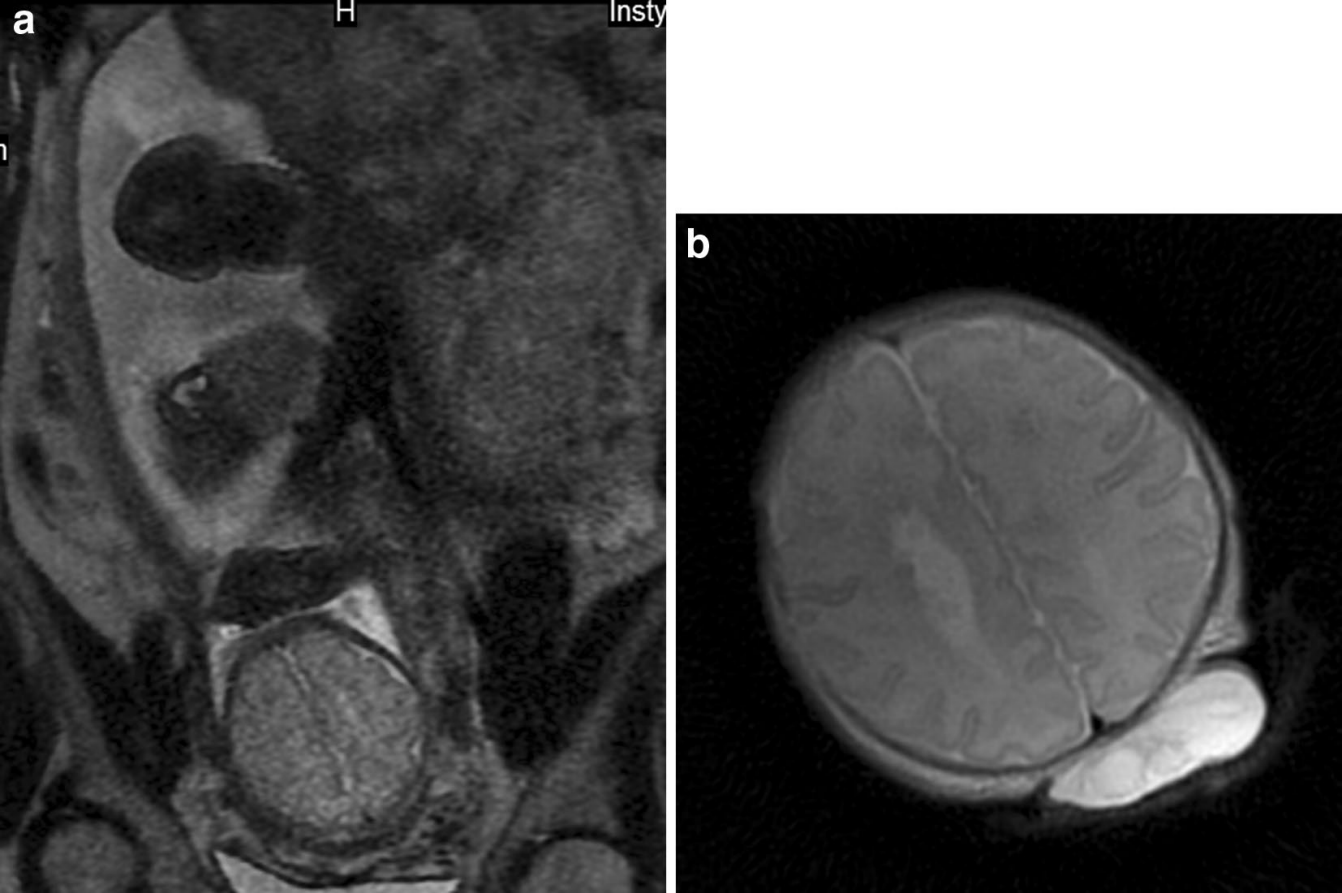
Case no. 3. a Prenatal MRI. T2-weighted image, axial plane. *Gray*
